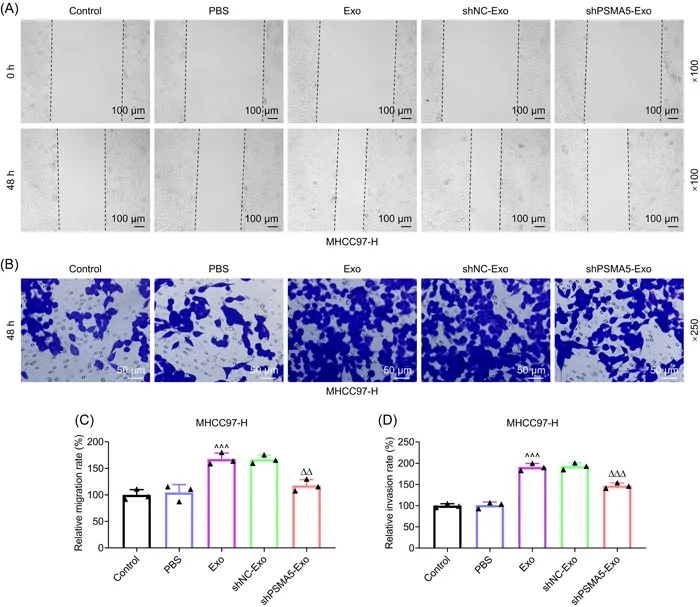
Knockdown of HCC cell‐secreted exosomal PSMA5 inhibited HCC migration and invasion induced by HCC cell‐secreted exosomes. (A, C) The migration of MHCC97‐H cells cocultured with THP‐1 cells under treatment of PBS or exosomes isolated from MHCC97‐H cells transfected with shNC/shPSMA5 or not was evaluated by wound healing assay (magnification: ×100; scale: 100 µm). (B, D) The invasion of MHCC97‐H cells cocultured with THP‐1 cells under treatment of PBS or exosomes isolated from MHCC97‐H cells transfected with shNC/shPSMA5 or not was evaluated by Transwell assay (magnification: ×250; scale: 50 µm). ^△△^
